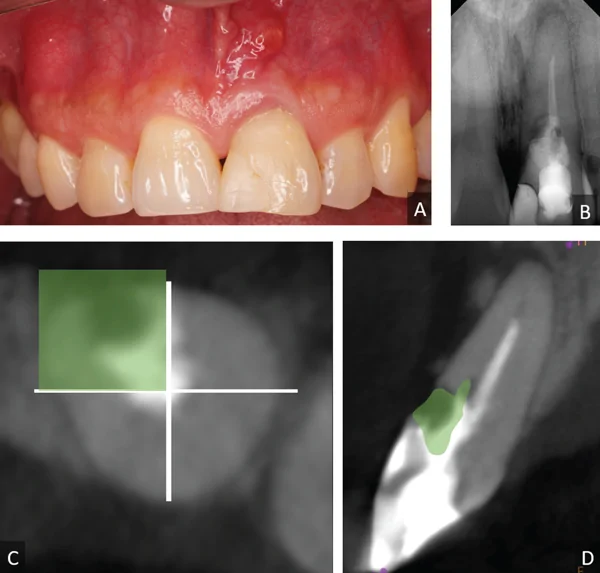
(A) Initial clinical presentation of the case. (B) Initial periapical radiographic image. (C,D) Axial and sagittal CBCT sections used to establish the classification according to the Patel [6] and Heithersay [4] criteria.
